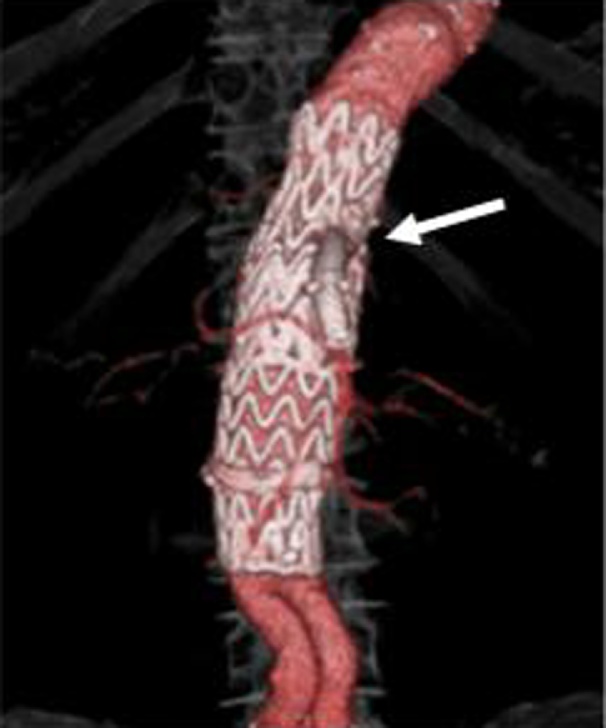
Abdominal computed tomography (CT) scan. Postoperative abdominal CT scan showed that endovascular aneurysm repair was performed and the superior mesenteric artery was reconstructed (white arrow).
